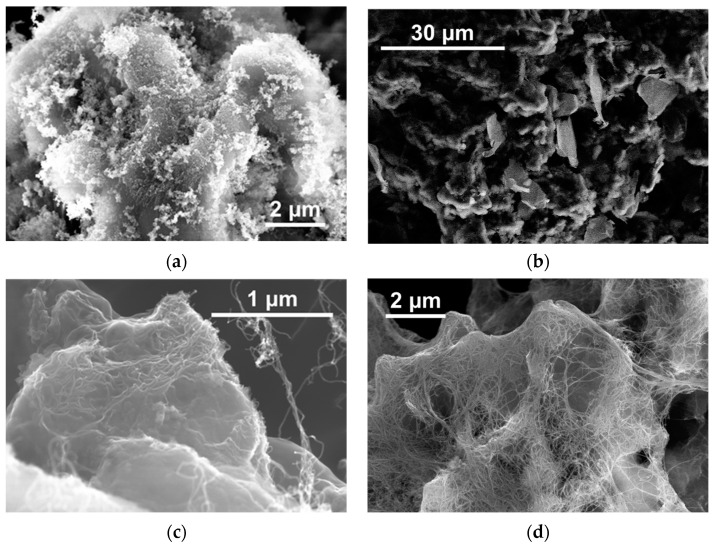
SEM images of the surfaces of the UHMWPE-1 RP after its ultrasound treatment in hexane in the presence of (a) 10 wt.% CB, (b) 10 wt.% GNPs, (c) 2 wt.% SWCNTs, or (d) 2 wt.% MWCNTs-1.
